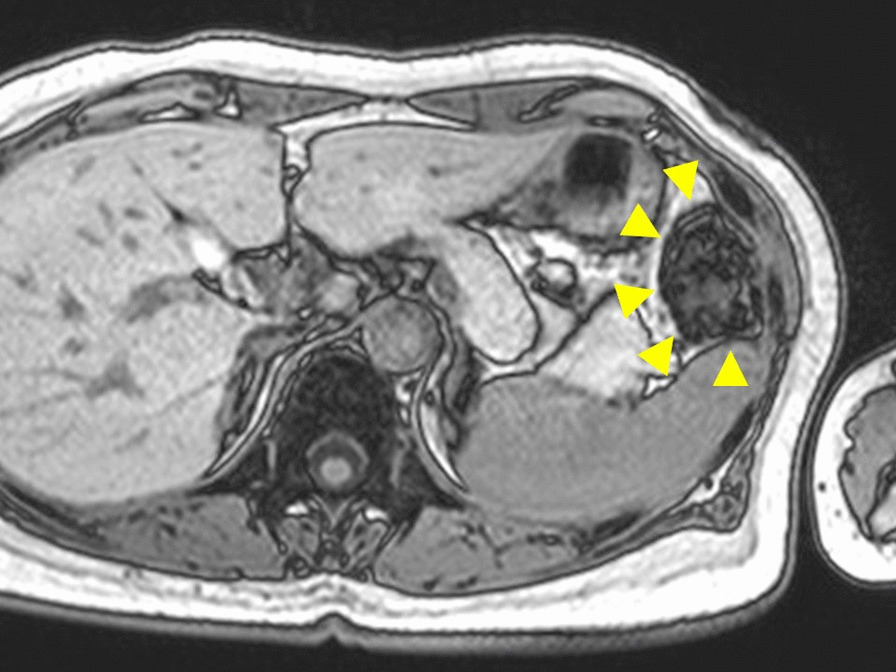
Magnetic resonance imaging of the tumor. Magnetic resonance imaging examination with contrast material shows a pedunculated tumor that attaches to the parietal peritoneum (arrowheads)
